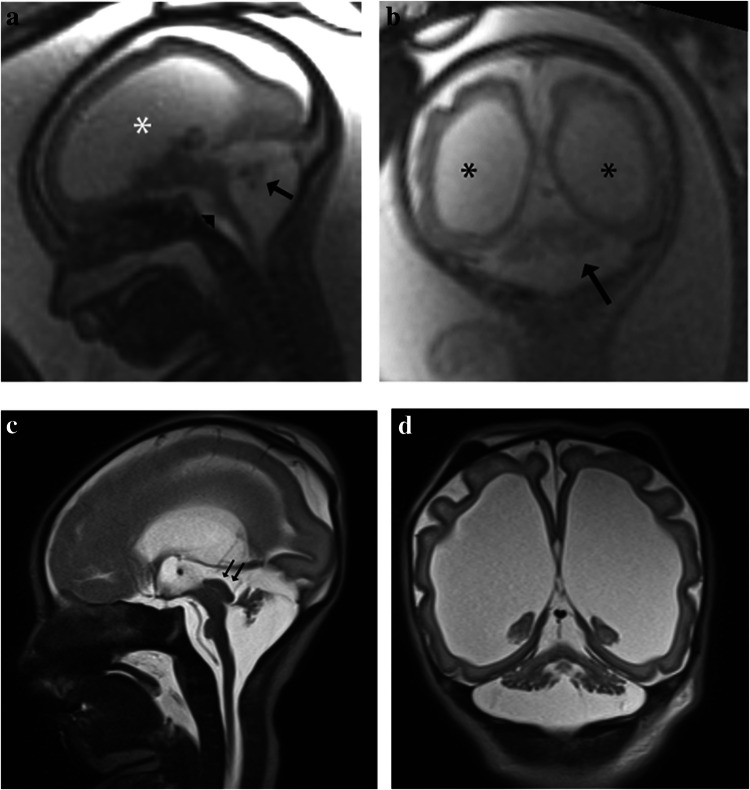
Pontocerebellar hypoplasia (type 4). Fetal MRI was performed at 32 1/7 weeks following ultrasound (*not shown*) documenting transverse cerebellar diameter <5th percentile, small vermis, and severe ventriculomegaly with cerebral mantle thinning. Sagittal (a) T2 fast steady-state image through the midline shows supratentorial ventriculomegaly (*asterisk*), severe flattening of the pons (*arrowhead*), and markedly small vermis (*arrow*). The absence of the corpus callosum (CC) versus pronounced thinning was unclear. Coronal (b) image through the posterior head shows the degree of parenchymal thinning and cerebellar hypoplasia. Postnatal brain MRI at 2 days of age, sagittal (c) and coronal (d) SSFSE images show the same findings as seen prenatally, with confirmation of absent CC and an additional note of tectal plate thickening (*double arrows*)
